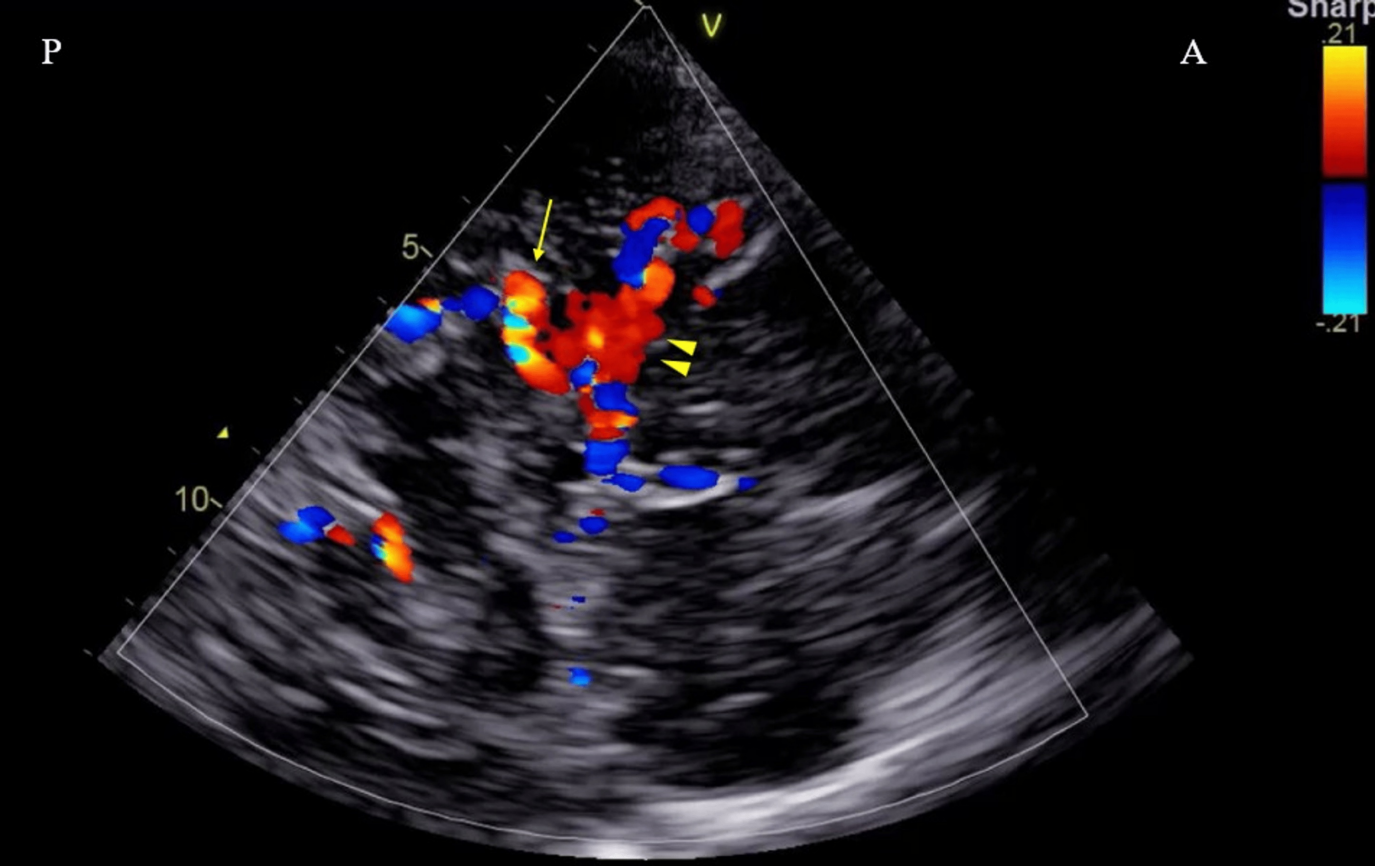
Transcranial color-coded duplex sonography (TCCS) through the right temporal window TCCS performed via the right temporal window through the postoperative bone defect created by prior superficial temporal artery to middle cerebral artery bypass surgery demonstrates moyamoya vessels (arrowhead) in the region corresponding to the abnormality identified on time-of-flight (TOF) magnetic resonance angiography. In addition, the right posterior cerebral artery is visualized (arrow). A represents the anterior aspect, and P represents the posterior aspect.
